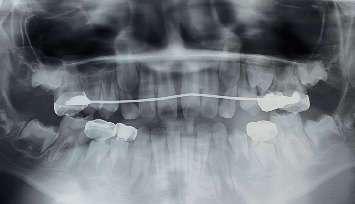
Radiographic control, Nance appliance (September 2019).
